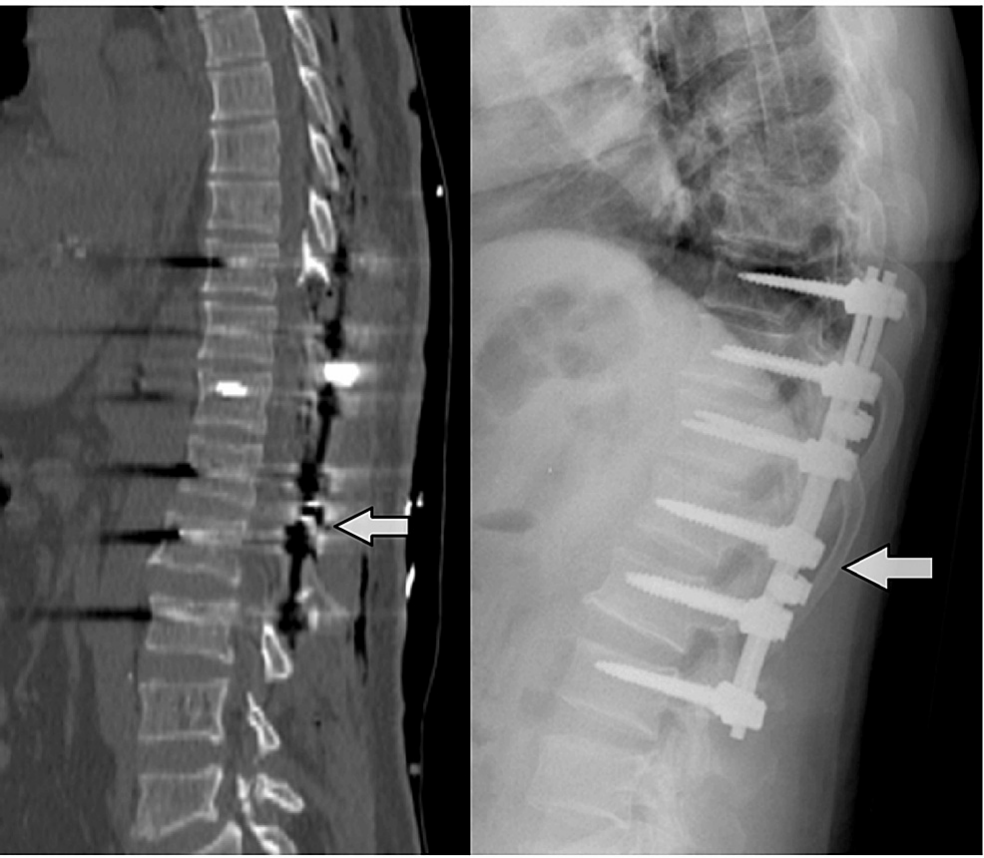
Postoperative images of the patient. A. Sagittal CT image because of five-level T10-L2 laminectomy. B. Lateral X-ray image showing T10-L3 posterior transpedicular stabilization. Arrows: operation field.
